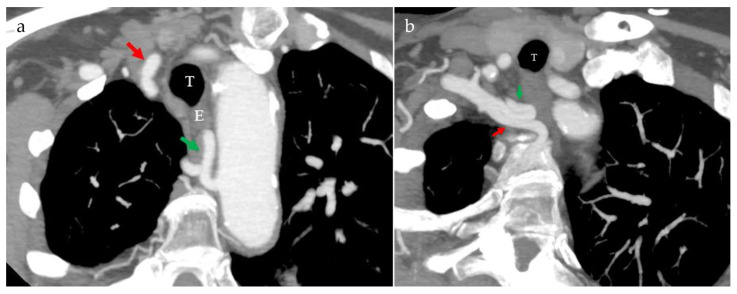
Maximum intensity projection images (a,b) of the CT scan show two arterial branches, the first one arising from the brachiocephalic trunk (red arrows) and the second one originating from the medial wall of the aortic arch (green arrows). Both vessels have a tortuous course, coiling around the esophagus and trachea, eventually merging to form the true right subclavian artery, which then follows its anatomical path. E = esophagus T = trachea.
